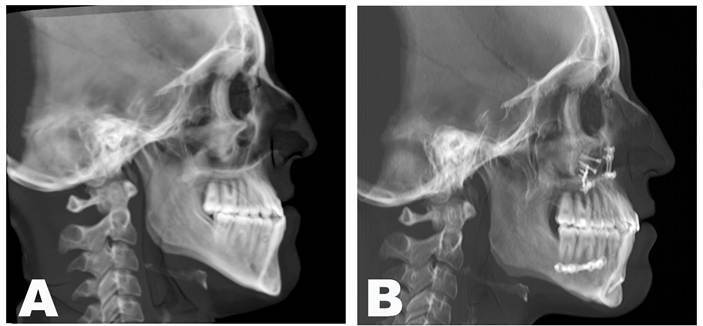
Lateral cephalometric radiograph: A) Preoperative and, B) Twenty-two months after the surgical procedure.
